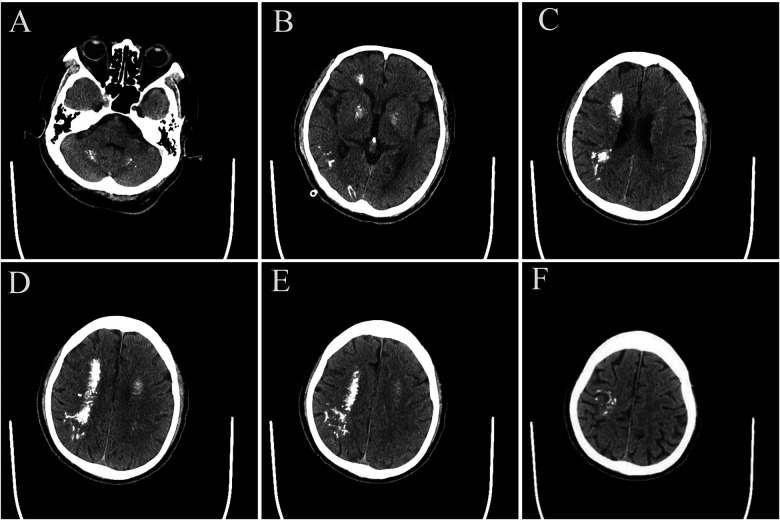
(A–F) cranial CT showed multiple calcified foci in the dentate nucleus region of both cerebellar hemispheres, the basal ganglia, the corona radiata region, and the right brain parenchyma, with the right cerebral hemisphere being the most prominent.
